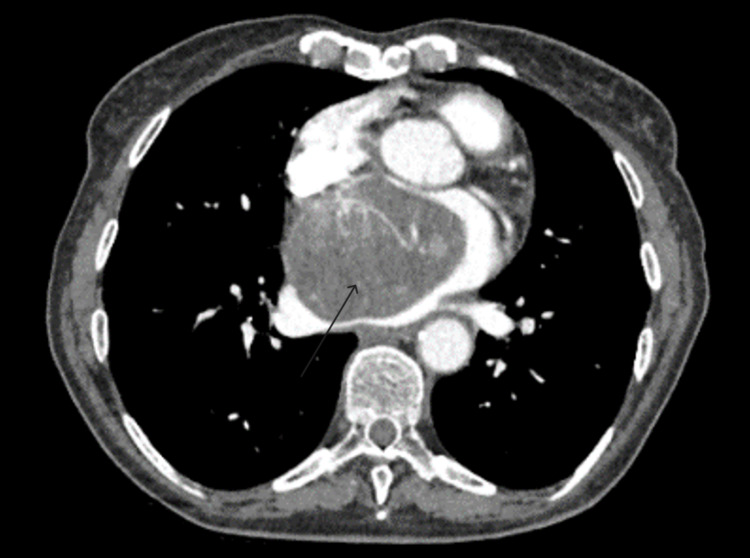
CTPA showing filling defect in the left atrium Arrow pointing to the atrial myxoma; CTPA: computed tomography pulmonary angiography
